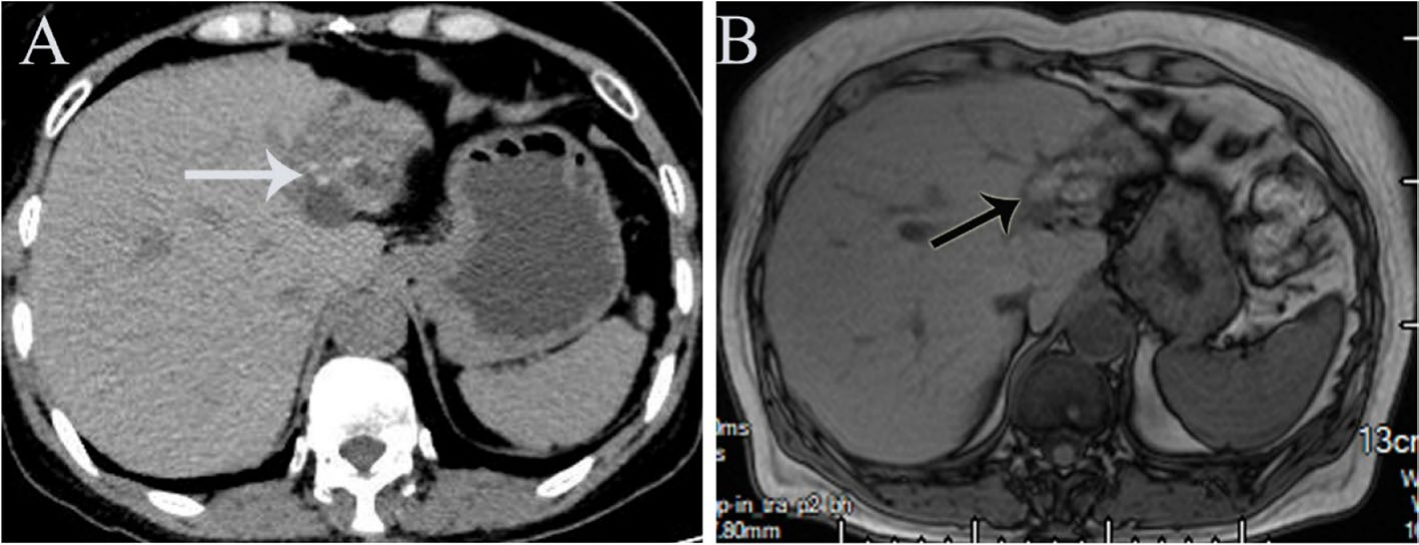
Preoperative imaging workup of left intrahepatic bile duct calculi and hepatic atrophy A the upper abdominal enhanced CT showing hepatolithiasis with dilatation of right-sided intrahepatic bile ducts (white arrow). B Contrast-enhanced MRI further delineated the anatomy of atrophy of the left lateral lobe of the liver (black arrow)
